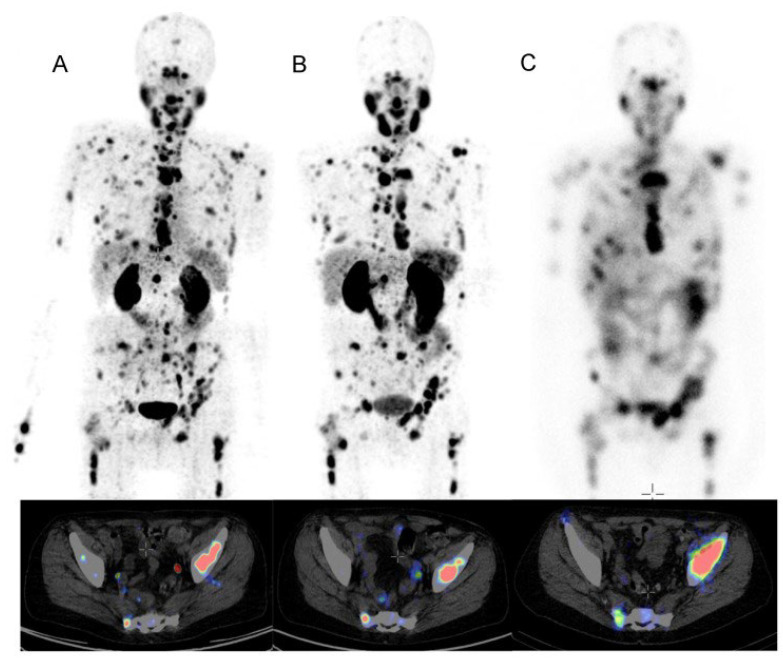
MIP (top) and slice (bottom) PET/CT of a 77-year-old mCRPC patient with high tumor load using (A) [^44^Sc]Sc-PSMA-617 (50 MBq, 60 min p.i.) and (B) [^68^Ga]Ga-PSMA-11 (120 MBq, 60 min p.i.); (C) planar scintigraphy (top) and SPECT/CT slice ~24 h after 6700 MBq [^177^Lu]Lu-PSMA-617 [32].
